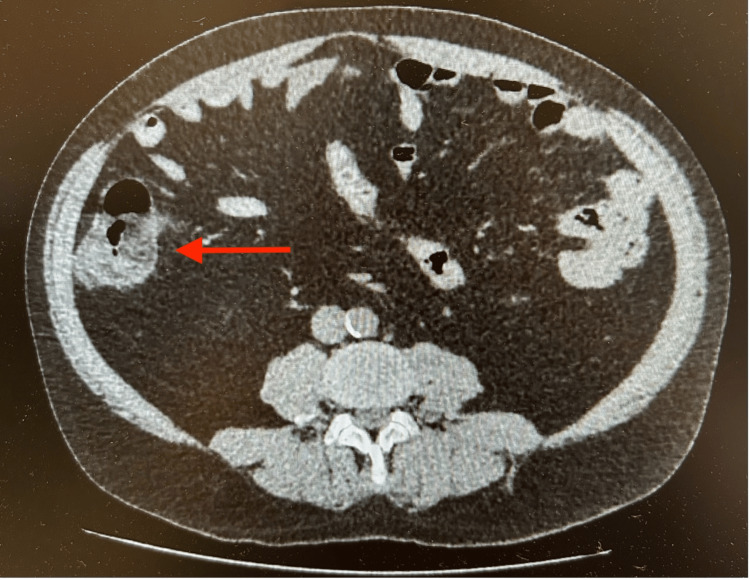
Contrast-enhanced computed tomography of the abdomen showing focal inflammatory involvement of the cecum (red arrow), with pericecal fat stranding.
